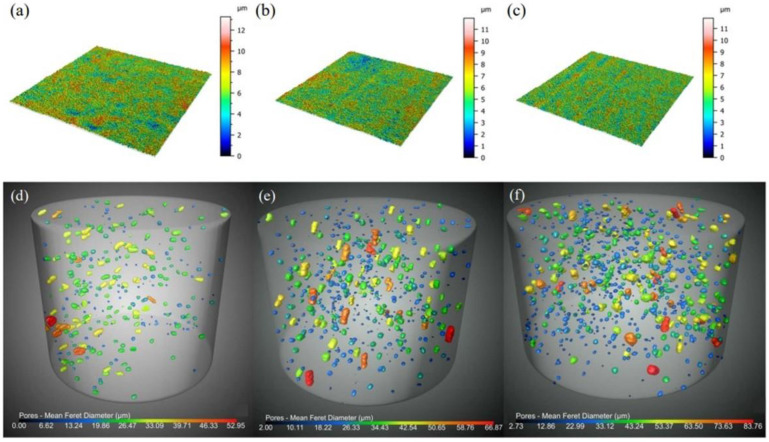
The surface morphologies of (a) ZF2, (b) ZF5 and (c) ZF10 sintered parts, and the computed tomography (CT) results of (d) ZF2, (e) ZF5 and (f) ZF10 sintered parts. (The scales in figures (d–f) are only applicable to micropores).
